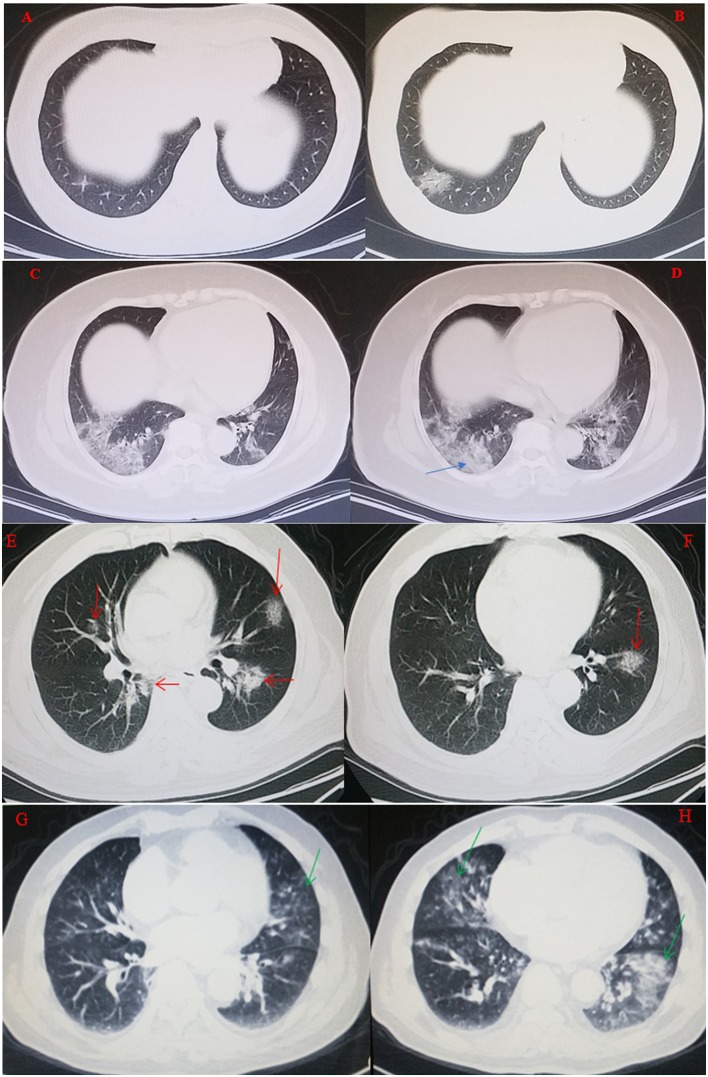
A 48-year-old woman visited the hospital due to fever, cough, and a history of traveling to Wuhan, China for 3 days. She underwent a chest CT scan on January 22, 2020, which showed a lesion pattern with the density of ground glass in the outside lung field of the right lower lung, (A). She was diagnosed with COVID-19 on January 23, 2020 and with a chest CT review performed on January 24, 2020, it can be seen that the original lesion pattern had progressed to a round state, (B). A 62-year-old woman visited the hospital due to cough, dyspnea, and fever for 4 days. There was no clear history of contact with an infected person. On January 28, 2019, a chest CT scan suggested a lesion pattern with the density of ground glass in the peripheral lungs, (C). She was diagnosed with influenza A pneumonia on January 30, 2019. Chest CT findings were reviewed again on January 31, at which point, the lesions had progressed with consolidations, as indicated by the arrows, and appeared patchy, (D). A 67 year old male patient with chest tightness and cough for 5 days, underwent lung plain CT scan on January 31, 2020. CT image: multiple ground glass density exudation shadow with grid shadow in both lungs, as the arrow points out (E,F) are CT images at the same day, with different scan levels. Nasopharyngeal swab for 2019-nCoV nucleic acid test positive. A 61-year-old male patient with a cough and fever for 3 days, on Febuary 1, 2019, lung pain scan CT was examined, ground glass density exudation shadow distributed in multiple parts of two lungs, as the arrow points out, (G,H) ae CT images at the same day, with different scan levels. Nasopharyngeal swab tested positive for influenza A antigen.
